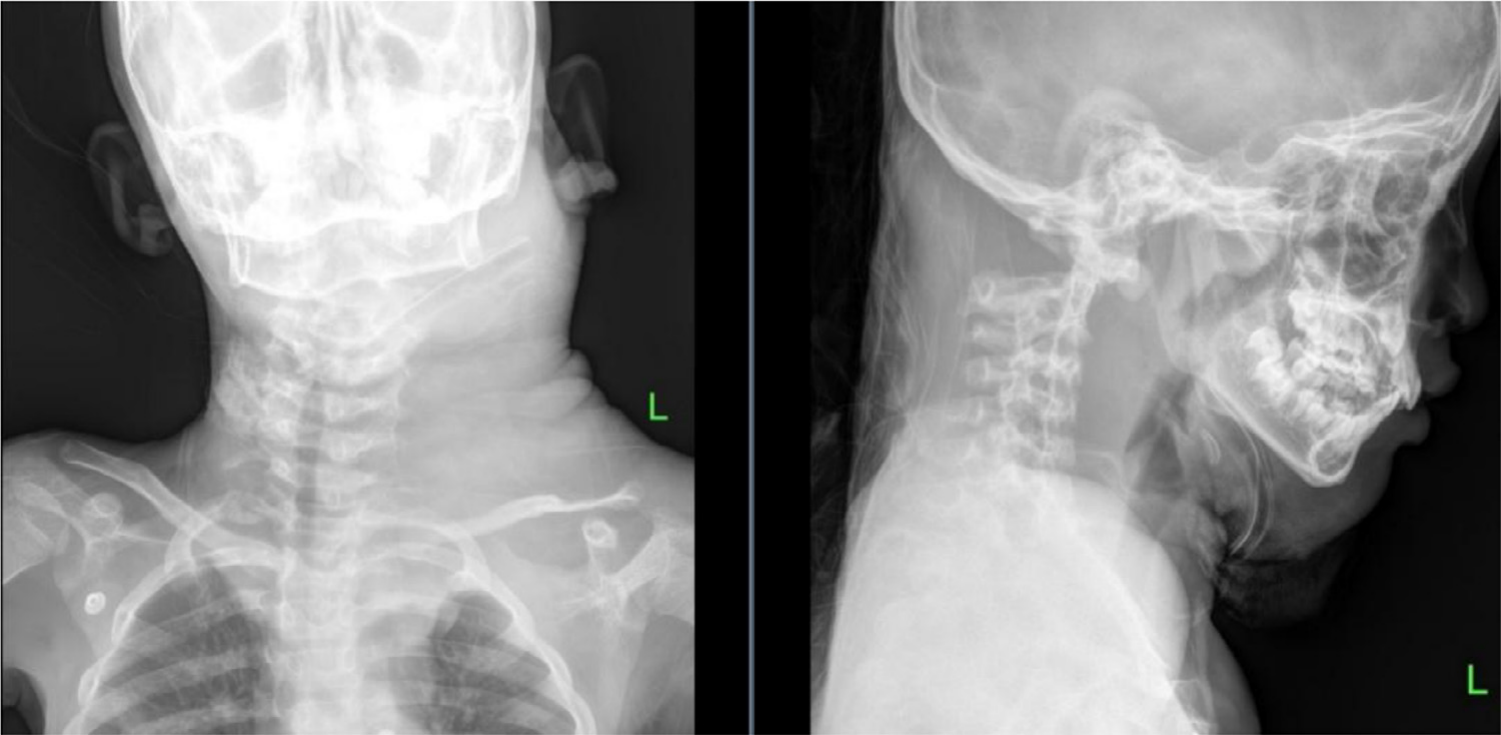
Cervical x-ray of the patient with plexiform neurofibroma.
